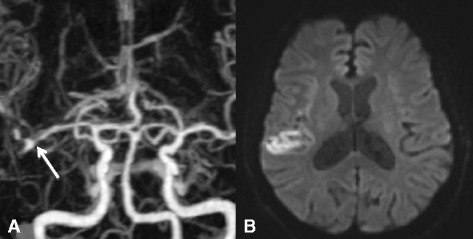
Noninvasive imaging. (A) Three-dimensional 360-slice CTA of the head of a 63-year-old woman presenting with headaches after a motor vehicle collision demonstrating a right M1 dissection with pseudoaneurysm immediately before the bifurcation (arrow); there is normal caliber of the contralateral MCA. (B) MRI brain shows diffusion restriction within the right perisylvian region consistent with acute ischemia. She was discharged on antiplatelet therapy with a modified Rankin Scale score of 0.
